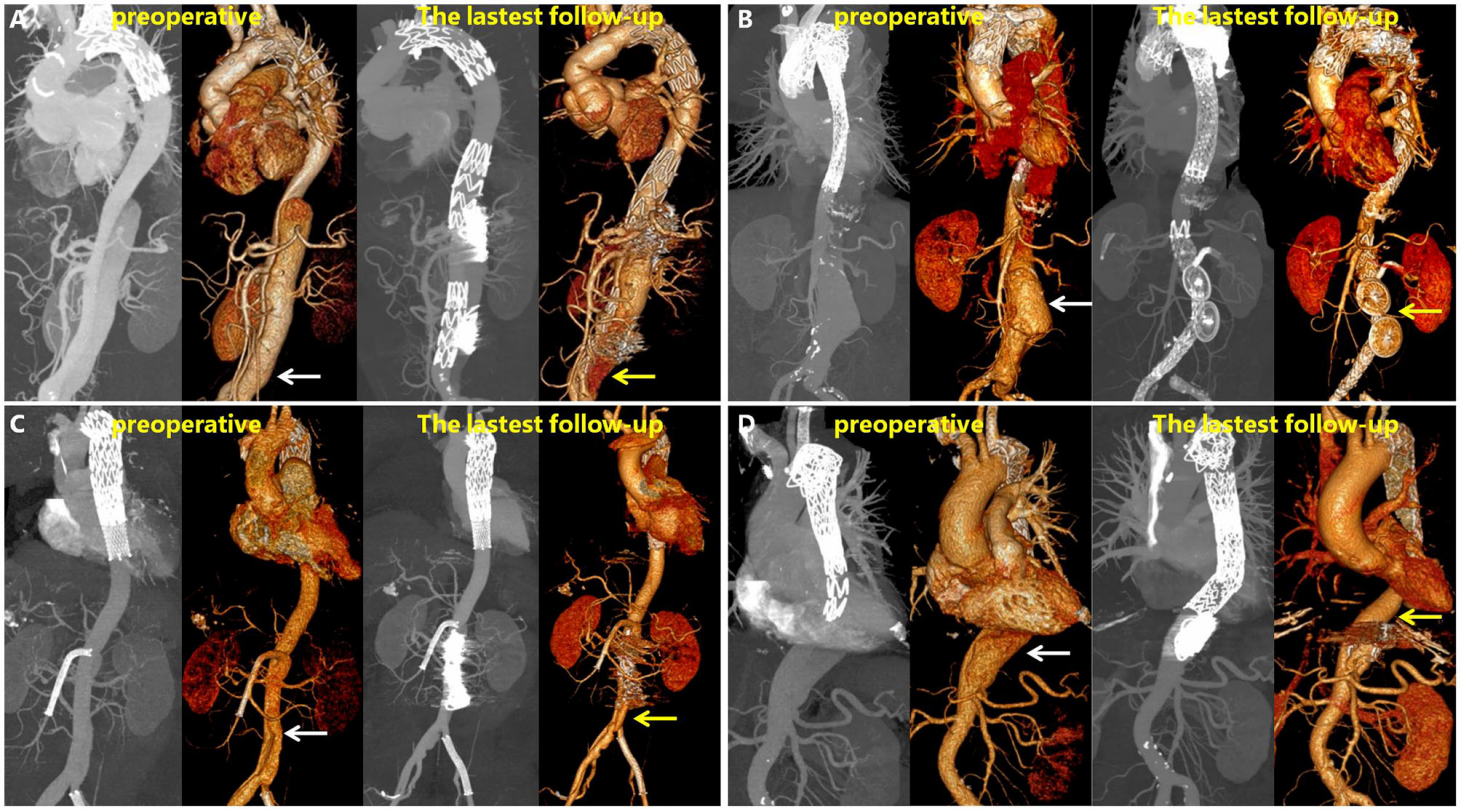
Maximum intensity projection (MIP) and 3D reconstructions of CTA in patients before and after RBS. White arrow points toward dilatation of the FL, and yellow arrow toward thrombosis formation in the FL or shrinking of the FL. (A) Patient's left renal artery was involved, and a slight endoleak was observed at 31 months. (B) Patient's left renal artery was involved, the FL disappeared at 43 months on 3D reconstruction images. (C) Patient's superior mesenteric artery was involved, the FL shrinked at 28 months. (D) Patient's celiac trunk was involved, the FL shrinked at 25 months.
